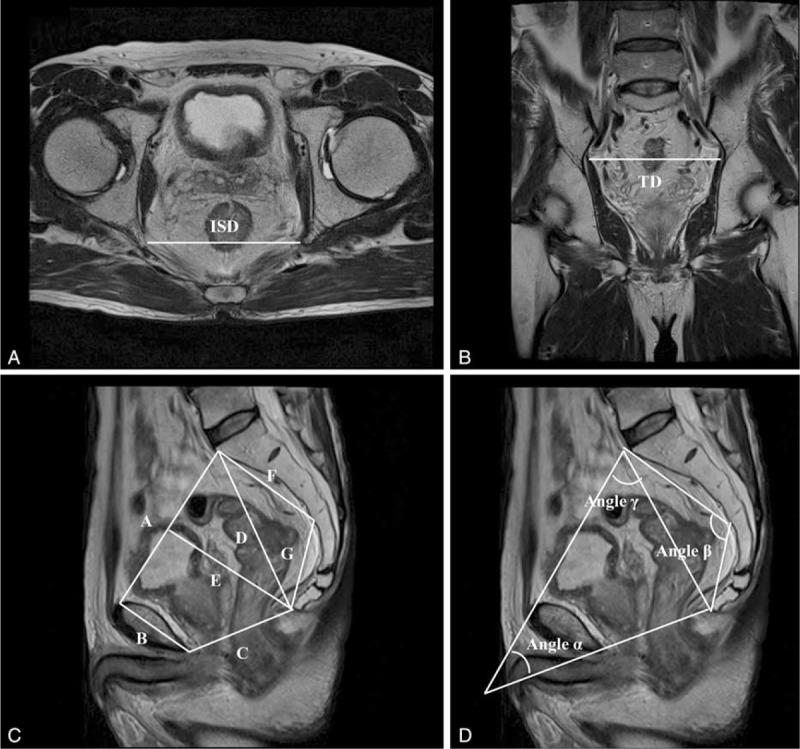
Pelvic dimension measurements in an (A) axial view, (B) coronal view, and (C, D) oblique view of pelvic magnetic resonance imaging.
